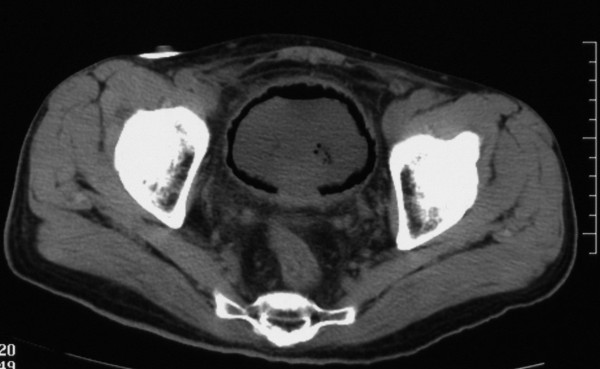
Computed tomography image showing extensive gas within the wall of the urinary bladder.
